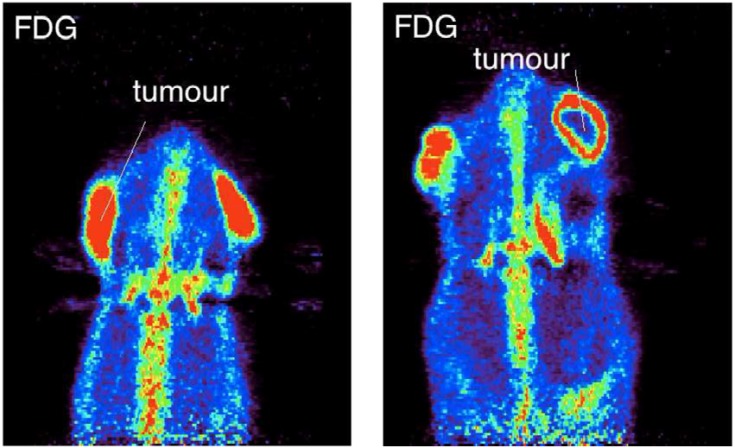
^18^F-FDG distribution in case of active tumor (left) and necrotic or hypoxic tumor (right).
